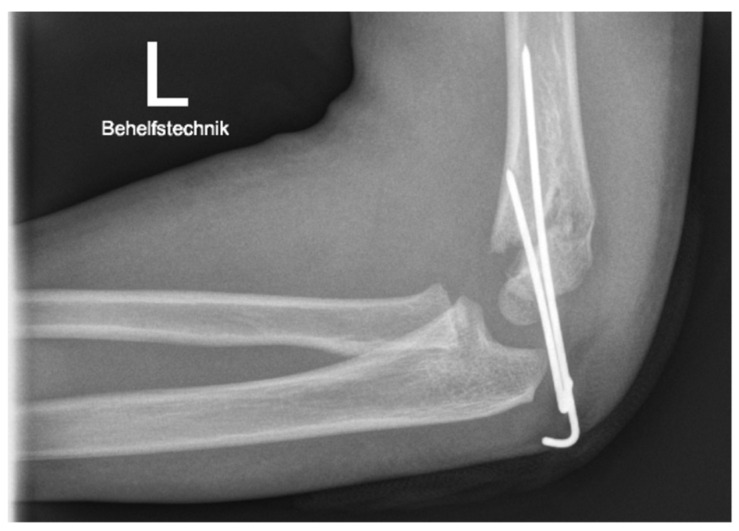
Postoperative radiograph of a rotational spur in the lateral view of a four-year-old patient after Gartland type 3 fracture and treatment by PCP.
